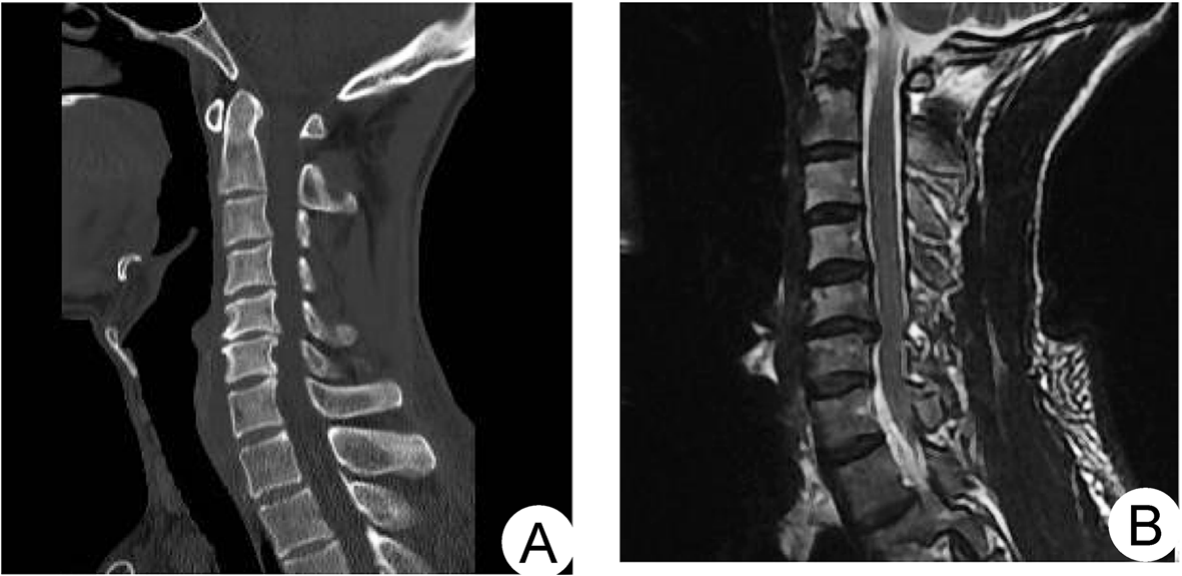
Preoperative CT images showed the formation of a disc-osteophyte complex in the cervical spine (A); preoperative MRI sagittal images showed significant compression of the spinal cord by the disc-osteophyte complex (B)
